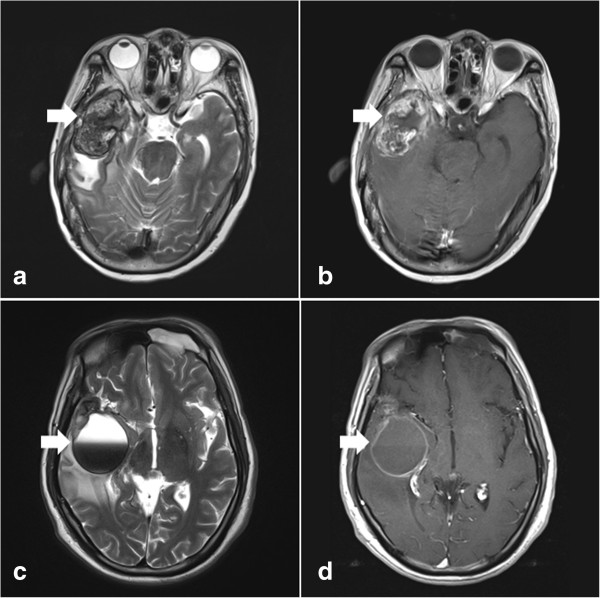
Radiation-induced temporal necrosis (RITN) presented on magnetic resonance (MR) images. RITN presented as a heterogeneously enhanced nodule (arrow in a: axial T2-weighted MR image; b: axial contrast-enhanced MR image) or cystic formation (arrow in c: axial T2-weighted MR image; d: axial contrast-enhanced MR image). Bleeding could be occasionally found in cystic RITN (arrowhead in c and d).
